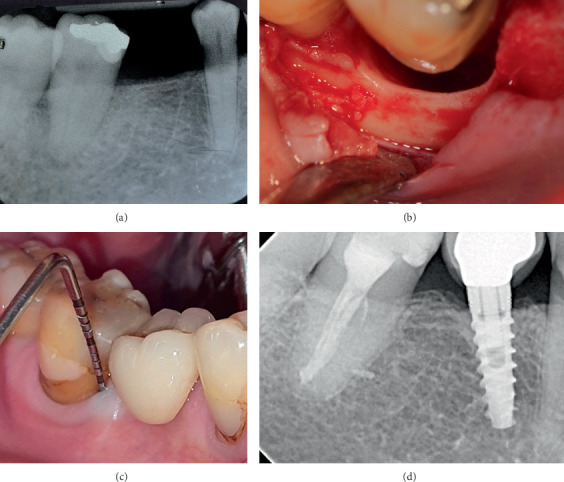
Clinical presentation of Case 3. (a) Baseline radiograph before surgery. A radiolucency at the apical–distal side of Tooth #47 can be noted. (b) A circumferential bone defect was observed on the buccal aspect of #47. (c) Thirteen-year follow-up: absence of periodontal inflammation and probing depth was reduced to 3 mm. (d) Radiograph at 13 years after surgery.
